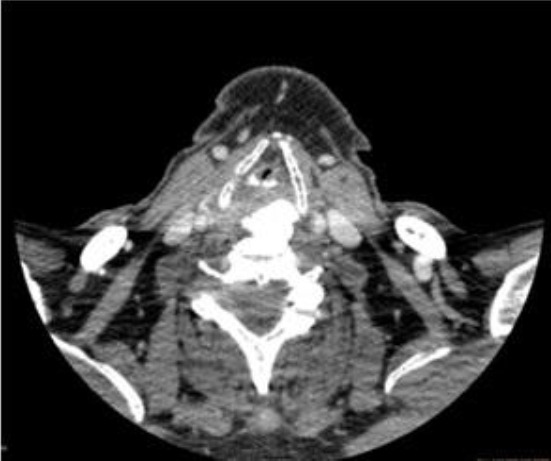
Computed tomography of the neck shows elongated ossification of the frontal planes of the vertebral body especially at right side*.
